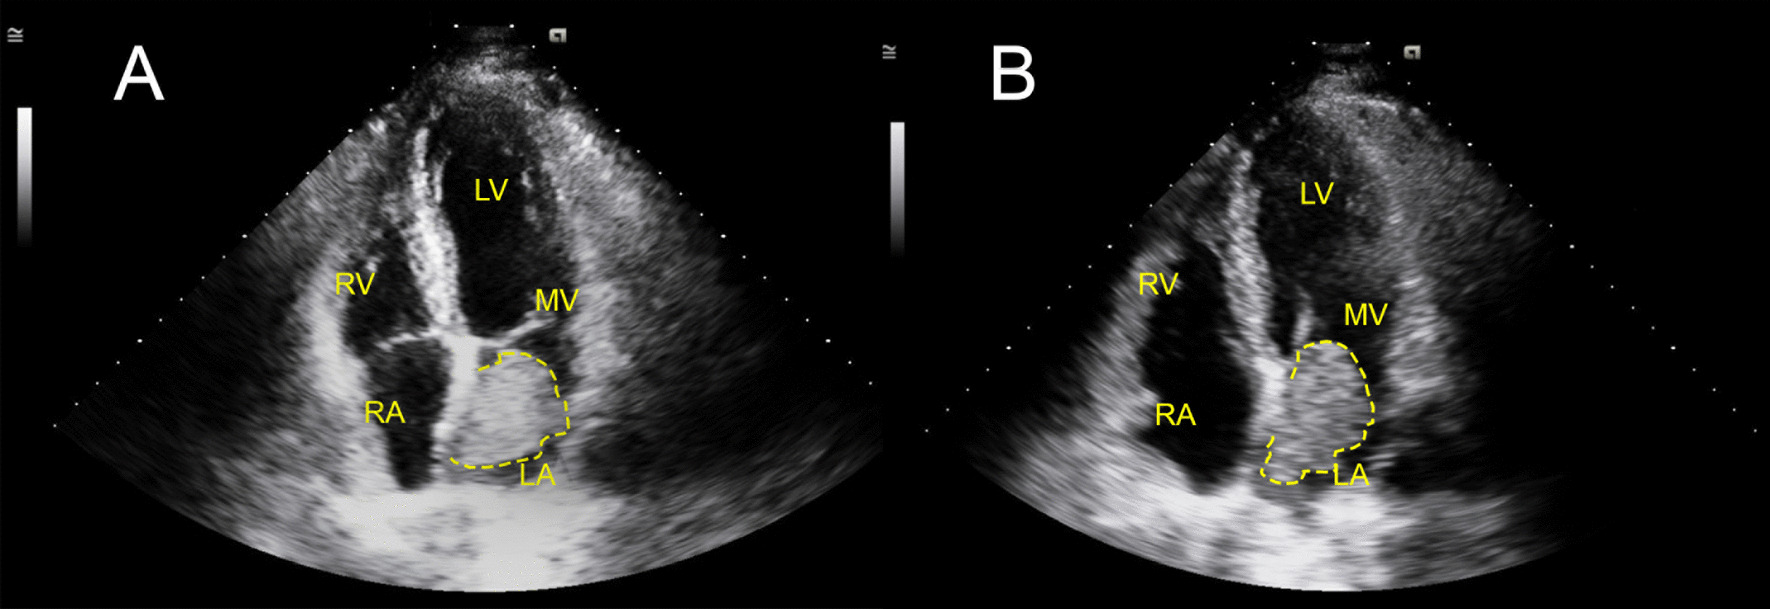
Transthoracic Echocardiogram During Systole and Diastole. a Left atrial myxoma attached to interatrial septum measuring approximately 3 cm × 4.8 cm. b Independently moving myxoma shown obstructing the mitral valve orifice during diastole. *LA* left atrium; *LV* left ventricle; *RA* right atrium; *RV* right ventricle
